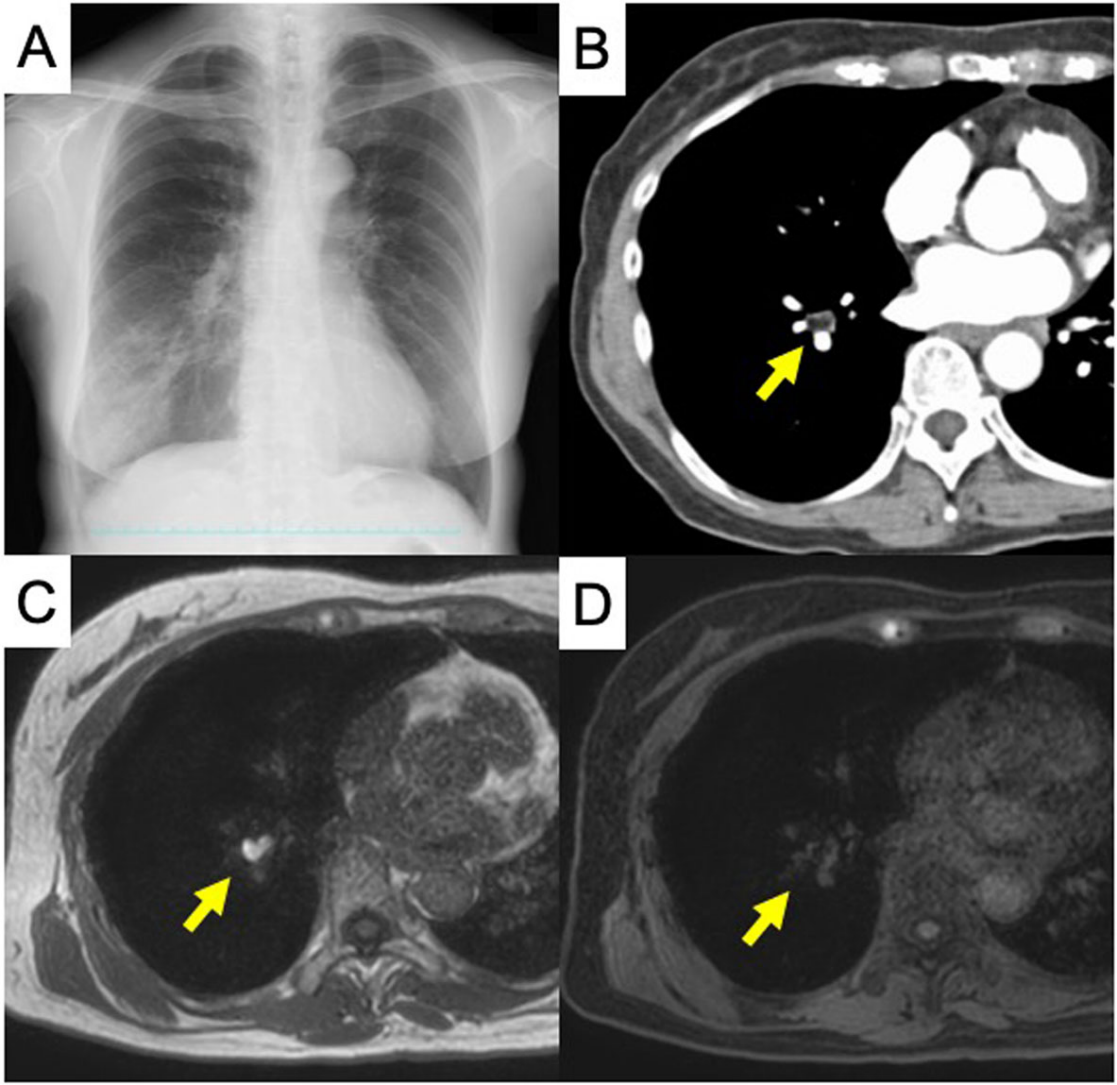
Preoperative imaging test. a Chest X-ray showing a shadow on the right lower lung field. b Computed tomography image showing a low-density nodule without calcification occluding the lumen of the lateral segmental bronchus of the right lower lobe (B^9^). c Magnetic resonance image showing a nodule of high intensity on T1-weighted images and d low intensity on fat suppression images
